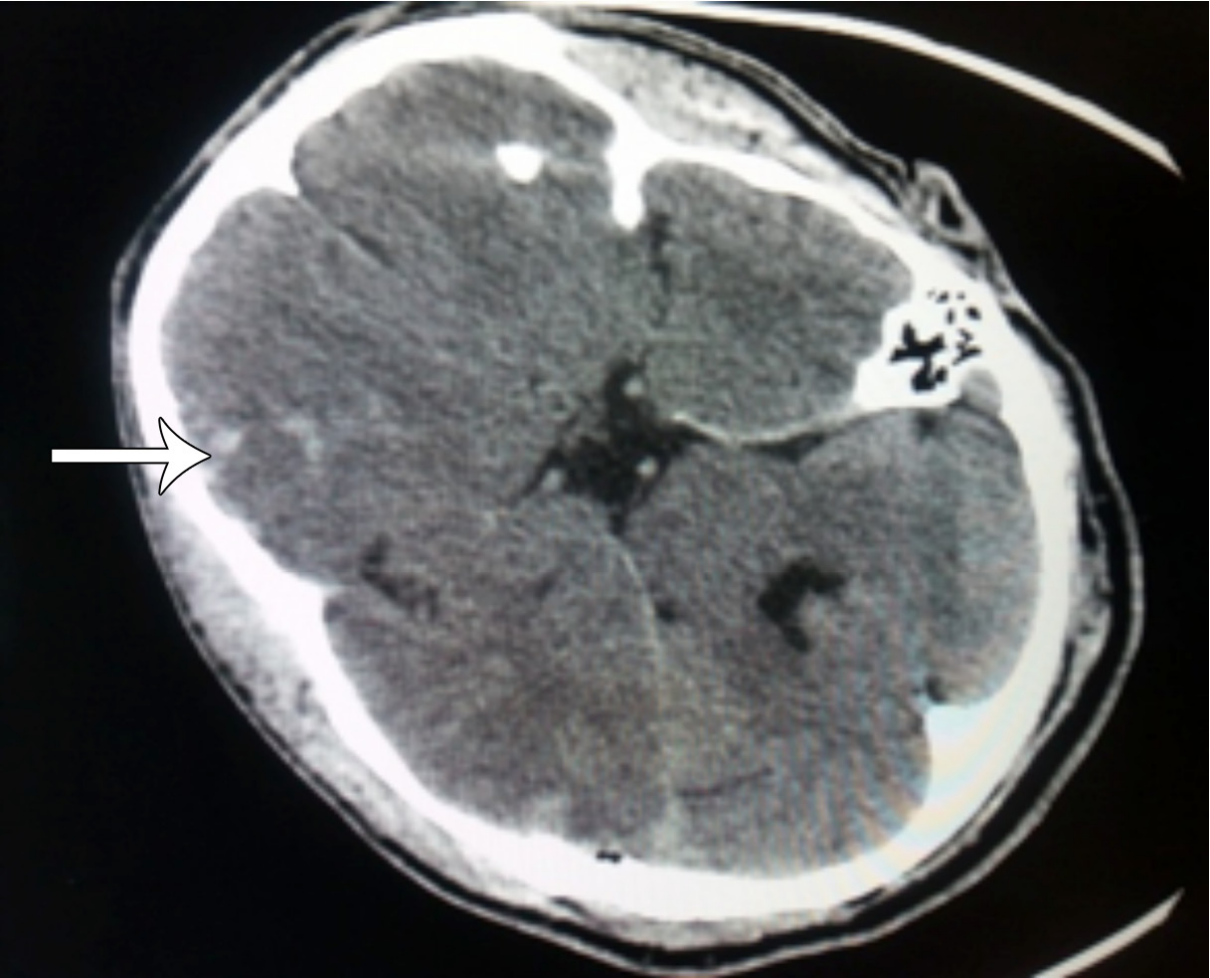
Computed tomography scan of brain at the time of admission (arrow).
